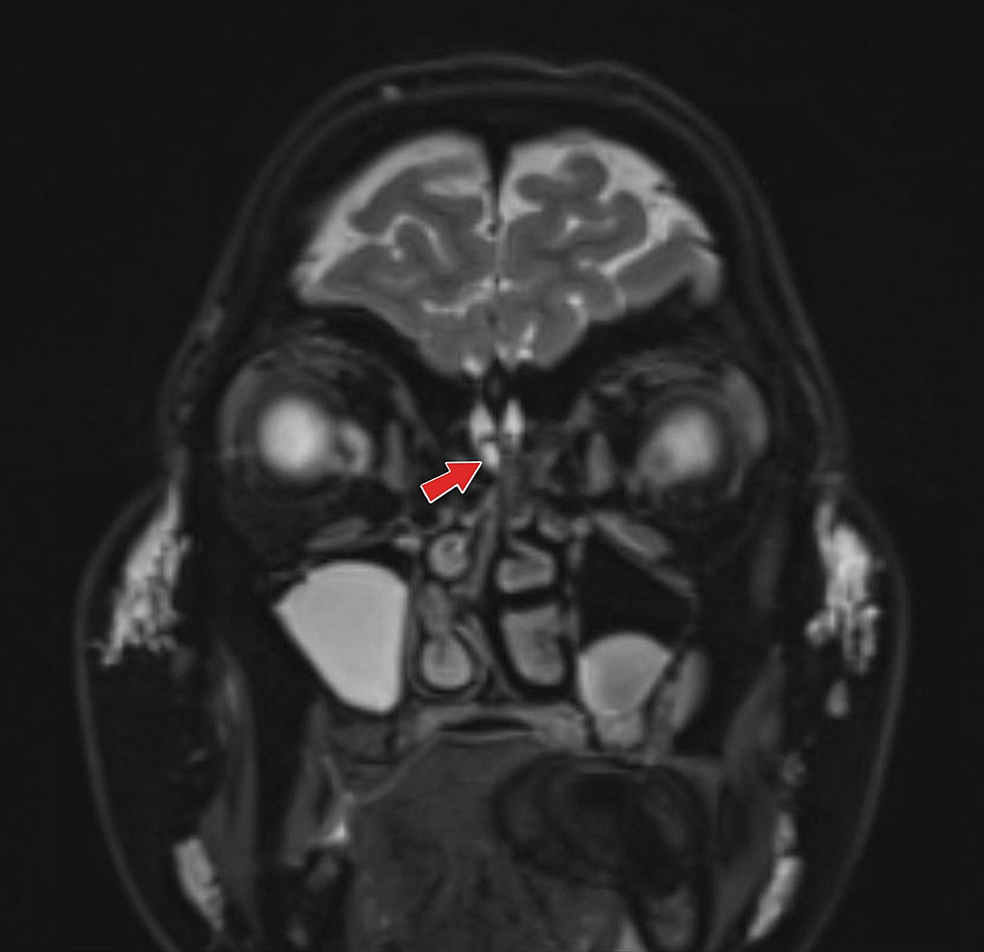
Magnetic Resonant Imaging (MRI) head with contrast showed a small amount of high signal intensity on the T2-weighted image (T2WI) seen infero-medial to the right olfactory bulb (red arrow), which suspected Cerebrospinal Fluid (CSF) leakage.
